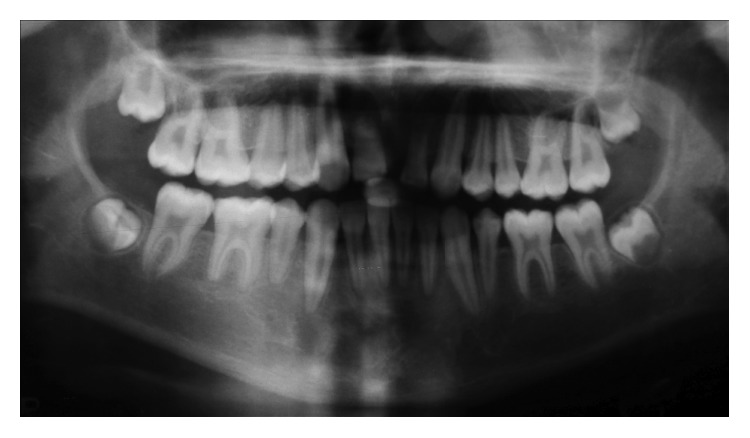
Radiographic view after orthodontic treatments.
